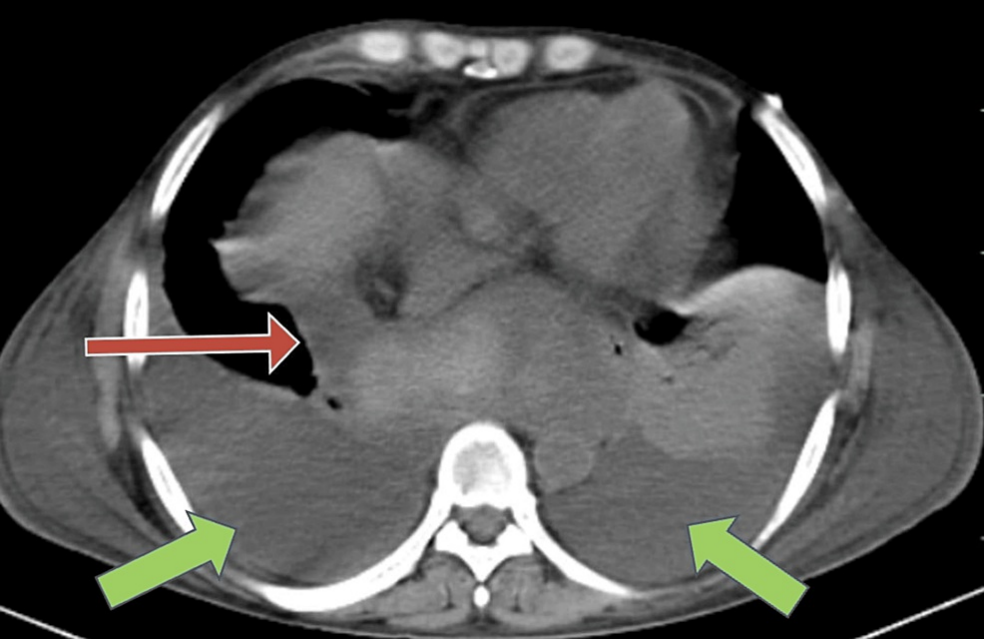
A pancreaticopleural fistula on the right side (red arrow) and bilateral pleural effusion (green arrows) were seen on the computed tomography scan of thorax
